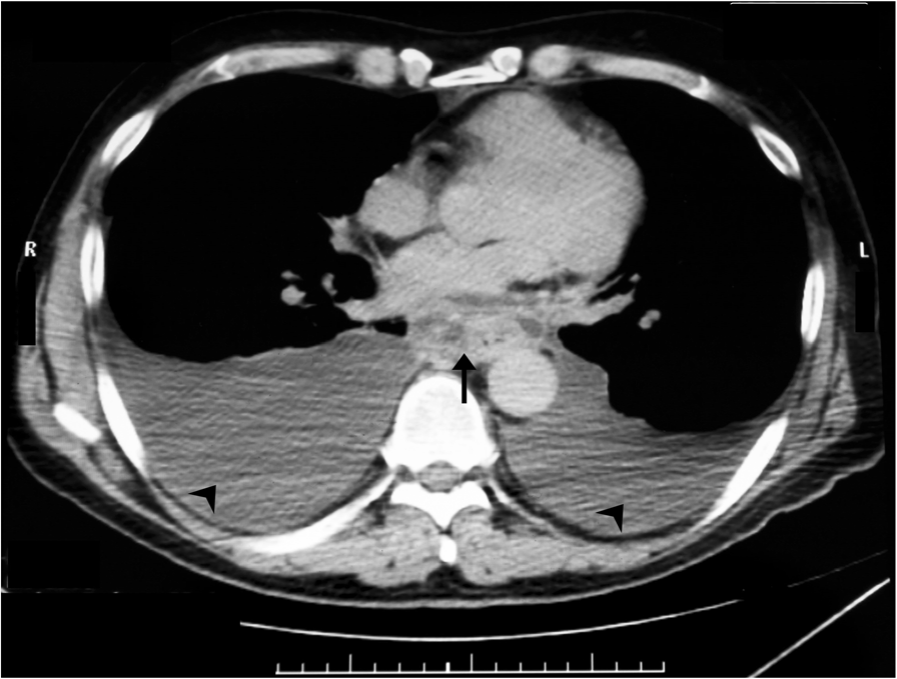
Axial post-contrast computed tomography scan at the level of the lower mediastinum reveals a mass (arrow) and bilateral pleural effusions (arrow heads).
